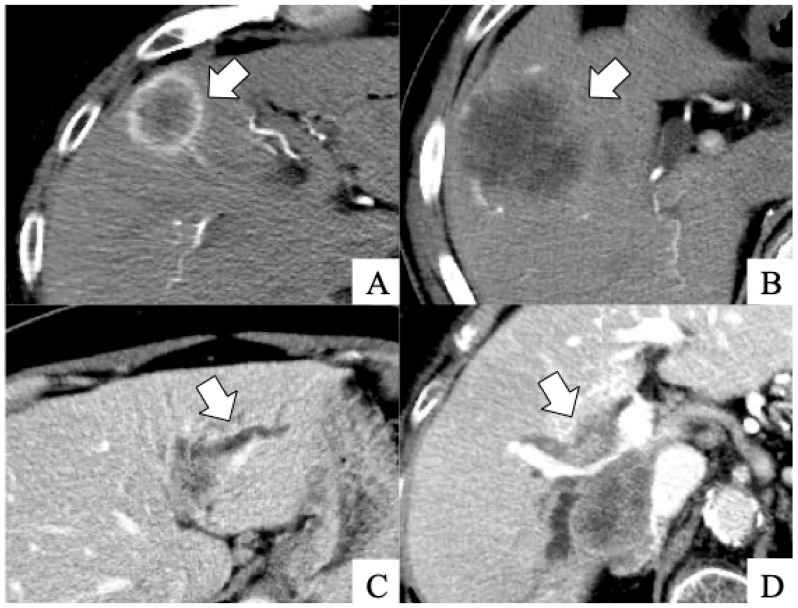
Dynamic computed tomography scan findings. (A) Rim-type enhancement in the early phase (arrow). (B) Absence of tumor enhancement in the early phase (arrow). (C) Peripheral biliary dilatation due to tumor invasion (arrow). (D) Penetrating Glisson’s vessel in the tumor (arrow).
